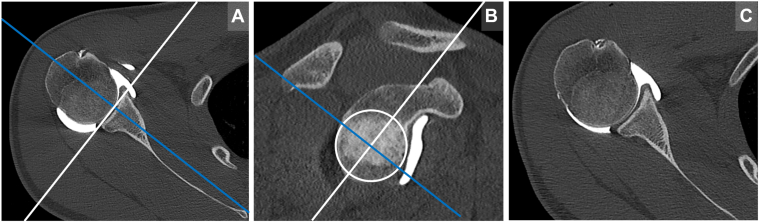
3D multiplanar reconstruction (MPR) of CT-imaging for standardized slice orientation and measurement reproducibility. (A) By adjusting the axial view axes perpendicular to the glenoid face and parallel to the scapular body, a strict sagittal plane aligned to the glenoid articular surface was selected. (B) In this sagittal plane, a best-fit circle was applied to the contour of the inferior glenoid. A line through the center of this circle and the long axis (*white line*) of the glenoid was determined. The plane perpendicular to this axis defined the standardized axial imaging plane (SAIP; *blue line*). (C) The axial CT slice in the SAIP used for subsequent measurements. *3D*, three-dimensional; *CT*, computed tomography; *SAIP*, standardized axial imaging plane.
